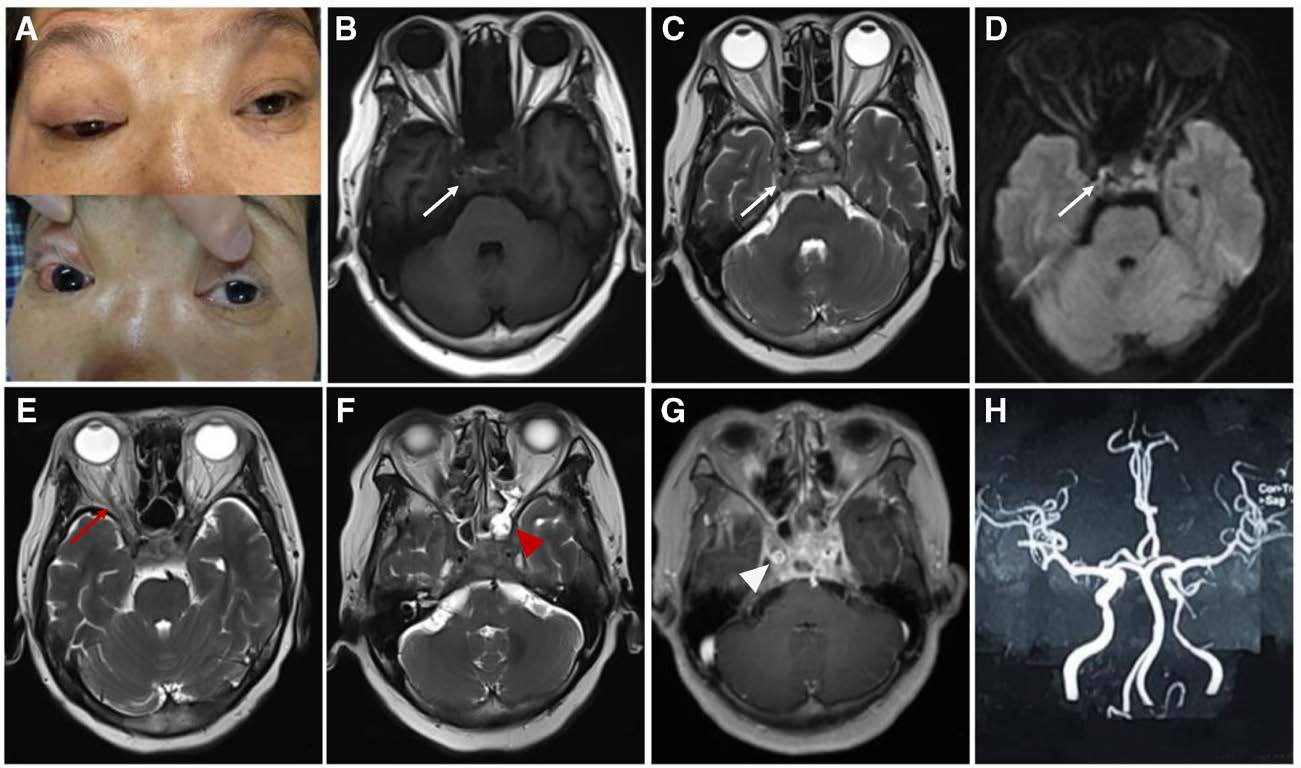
On admission, neurological examination revealed bilateral ptosis and right exophthalmos and conjunctival congestion (A). On day 3 after admission, brain MRI showed mixed signal intensities in both cavernous sinuses on T1-weighted, T2-weighted and diffusion-weighted images (white arrows) (B-D). Axial T2-weighted images revealed expansion of right superior ophthalmic vein (red arrow) and sphenoid and ethmoid sinusitis (red arrowhead) (E, F). Axial Gadolinium enhanced-T1-weighted image demonstrated expansion of both cavernous sinuses with circular enhancement of the arterial walls (white arrowhead) (G). MRA disclosed stenosis of the left ICCA (H). ICCA = intracavernous carotid artery, MRA = magnetic resonance angiography, MRI = magnetic resonance imaging.
